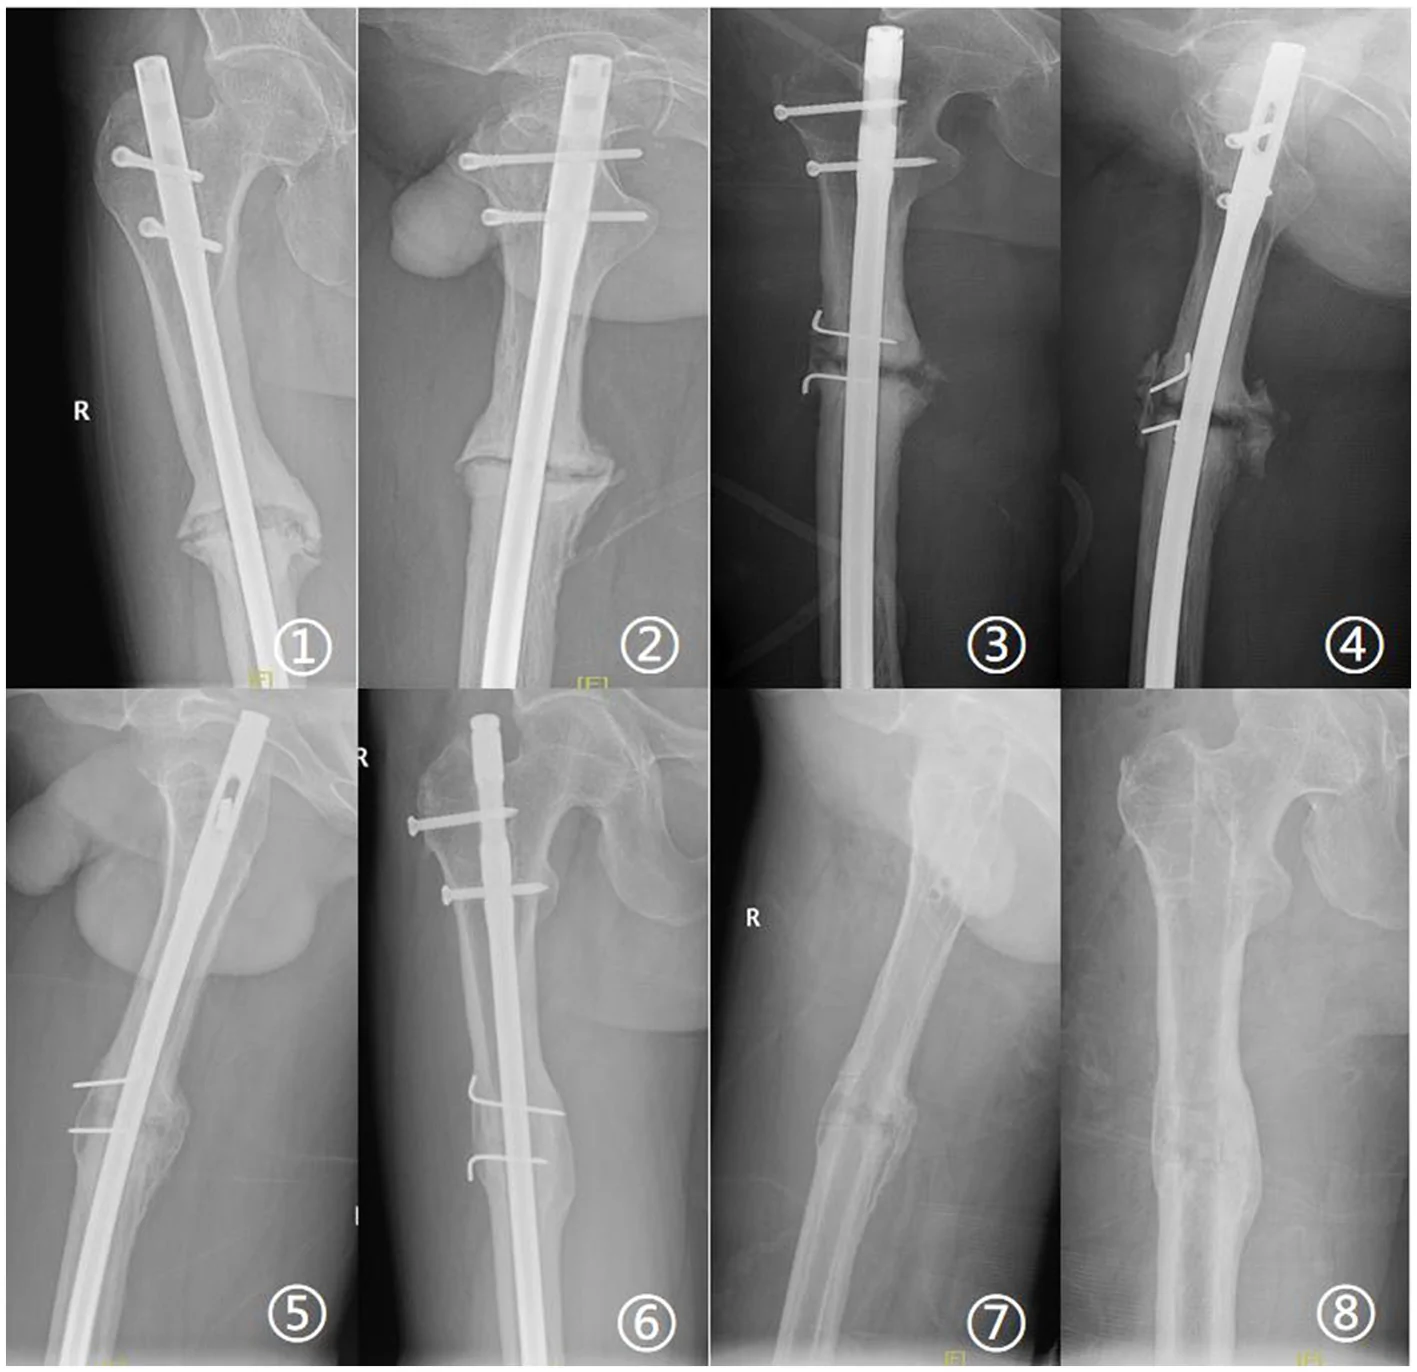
Minimally invasive elastic Poller wire augmentation for hypertrophic femoral non-union. (1, 2) Pre-operative AP and lateral radiographs showing hypertrophic non-union. The IMN remains intact, but sclerotic margins and abundant callus are present due to micromotion. (3, 4) Immediate post-operative radiographs. Two 2.5 mm K-wires were inserted transcortically via a 3–5 cm mini-incision. The wires lay tightly against the IMN and penetrated both cortices, acting as wedges to eliminate the micromotion (“windshield wiper effect”) of the IMN. The wire tails were bent to prevent intra-medullary migration and to facilitate future minimally invasive removal. (5, 6) Radiographs at 3 months showing an improved stress environment with massive bridging callus. (7, 8) Radiographs at 6 months showing complete union and partial recanalization of the medullary cavity after the K-wires were removed via a minimally invasive approach under local anesthesia, demonstrating excellent biological preservation.
